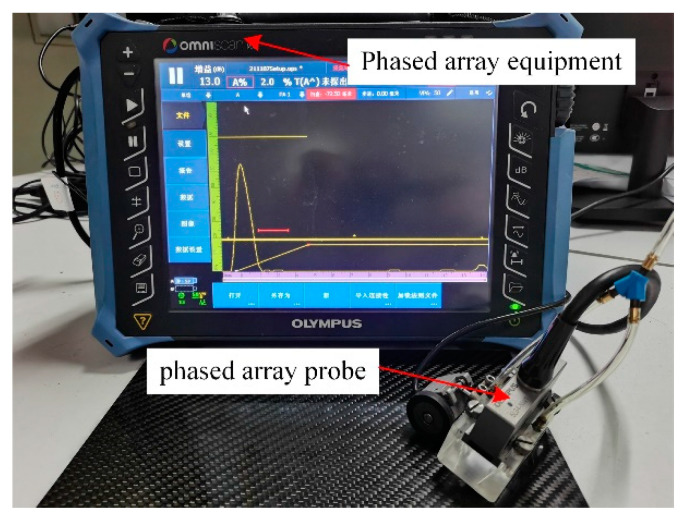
Ultrasonic phased array testing equipment.
